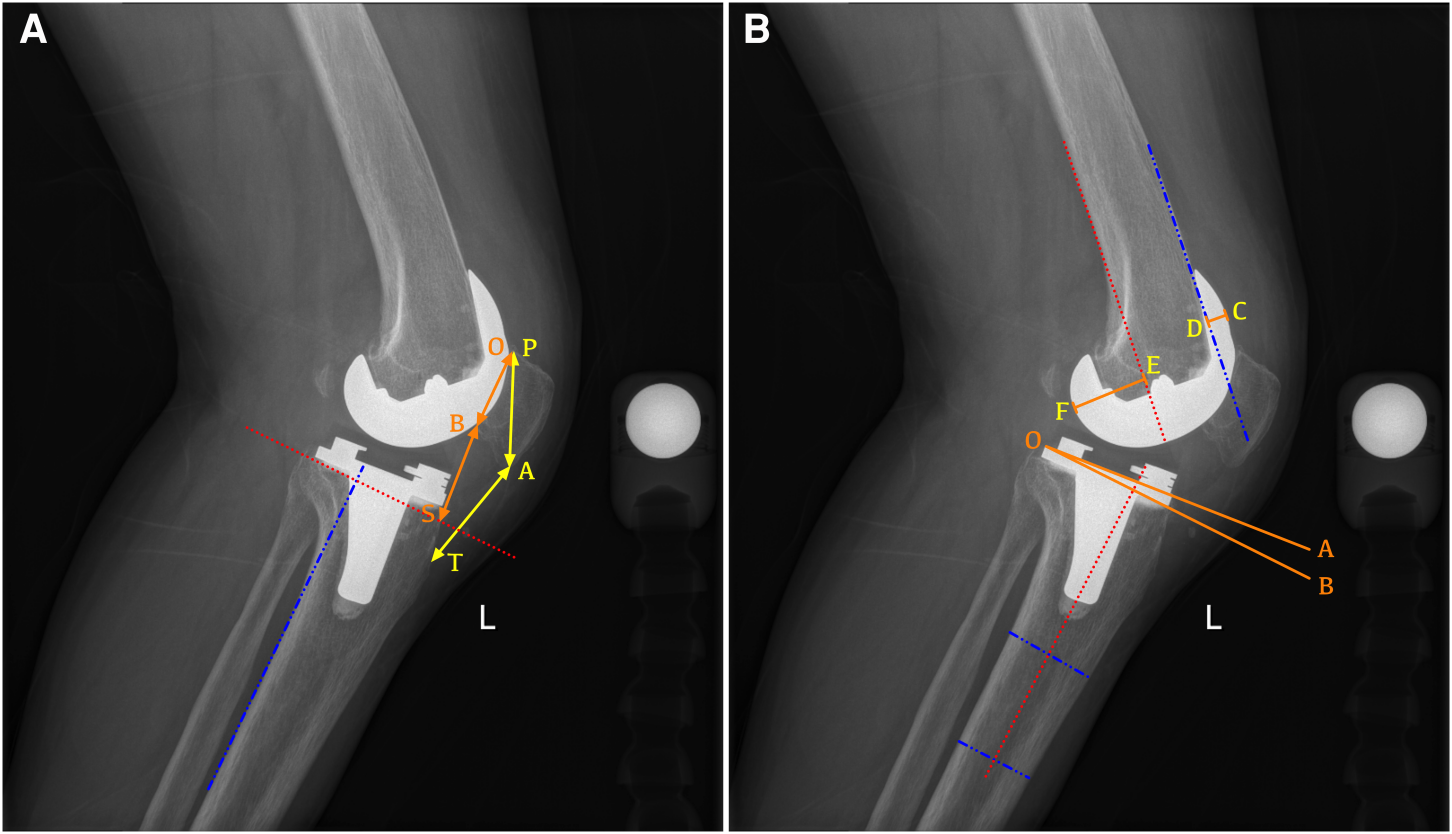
Radiographic measurement of the lateral view after PS-TKA. (A) Example of a radiograph demonstrating how to measure the Insall–Salvati ratio (AT/AP) and modified Caton–Deschamps ratio (BS/BO). AT, length of the patellar tendon; AP, longest diagonal length of the patella; B0, length of the articular surface of the patella; BS, distance from the distal portion of the articular surface of the patella (B) to the intersection between the line perpendicular to the tibial posterior cortex elevated at the tip of the fibular head and the tibial anterior cortex (S). (B) Example of a radiograph demonstrating how to measure the proximal anatomic axis posterior tibial slope (the angle between lines AO and BO), the anterior condylar offset (CD, the distance between the anterior femoral cortical margin and the anterior margin of the femoral condyles), and posterior condylar offset (EF, the distance between the posterior femoral cortical margin and the posterior margin of the femoral condyles).
